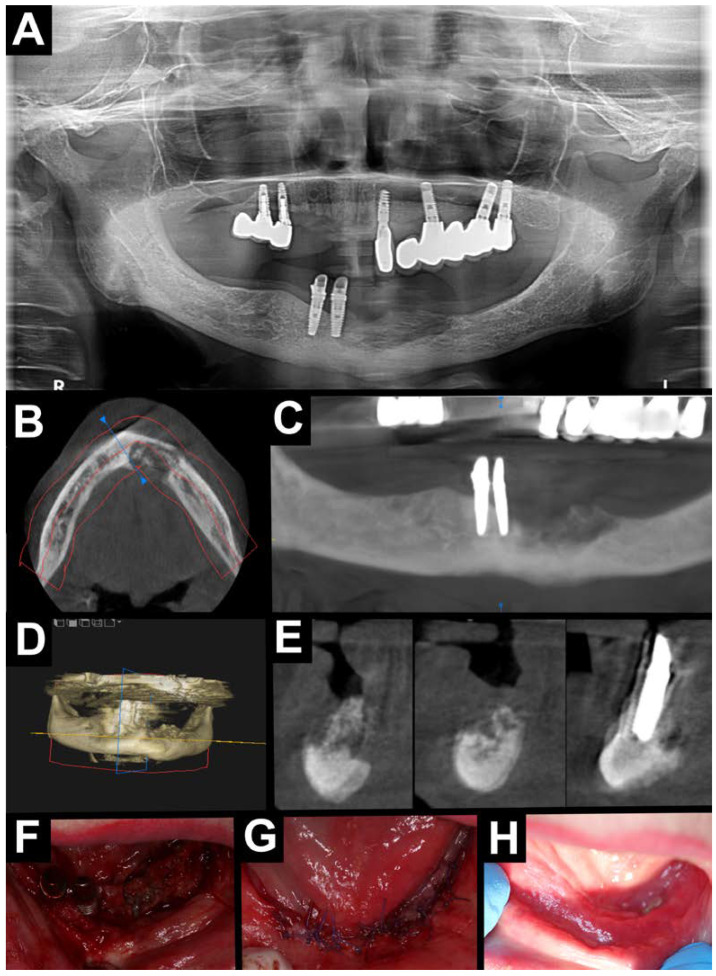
Treatment without A-PRF. Radiological diagnostic imaging of MRONJ (A–E). (A) Orthopantomography—area with osteolysis and osteosclerosis located in the mandible on the anterior area; CBCT (B–E). (B) Axial view—area with osteolysis and osteosclerosis on the anterior area of the mandible. (C) Orthopantomographic reconstruction—area with osteolysis and osteosclerosis on the anterior area of the mandible. (D) Pseudo-3D reconstruction—area with osteolysis and osteosclerosis on the anterior area of the mandible. (E) Cross-sectional view—area with osteolysis and osteosclerosis on the anterior area of the mandible. Intraoral photographs (F–H). (F) Visible necrotic bone after mucoperiosteal flap elevation. (G) After suturing the wound without tension. (H) Healing after 2 weeks (red line—panoramic reconstruction site; yellow line – cross-sectional area line; blue line—cross-sectional area line).
